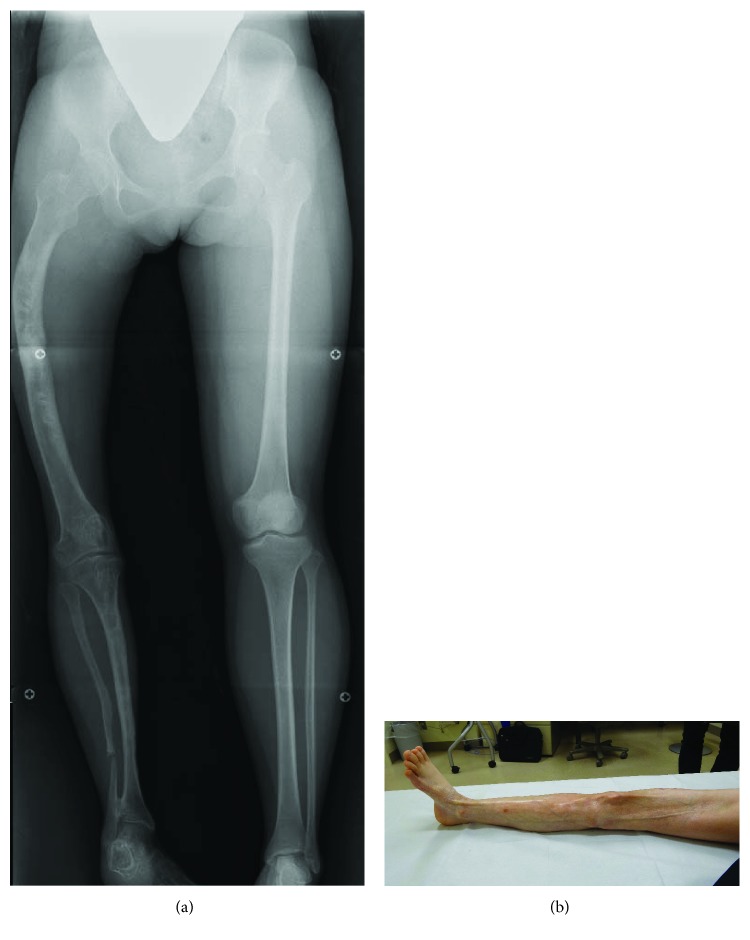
Current radiograph and view of the lower extremities. (a) An anteroposterior supine radiograph of the lower extremities at 17 years of age showing an anterolateral bowing of the right femur and a medial bowing of the right tibia. (b) A postoperative view of the right lower extremity showing atrophic skin surfaces on the posteromedial aspect of the thigh and the medial aspect of the lower leg, where brown pigmented cutaneous lesions were observed at the first presentation.
